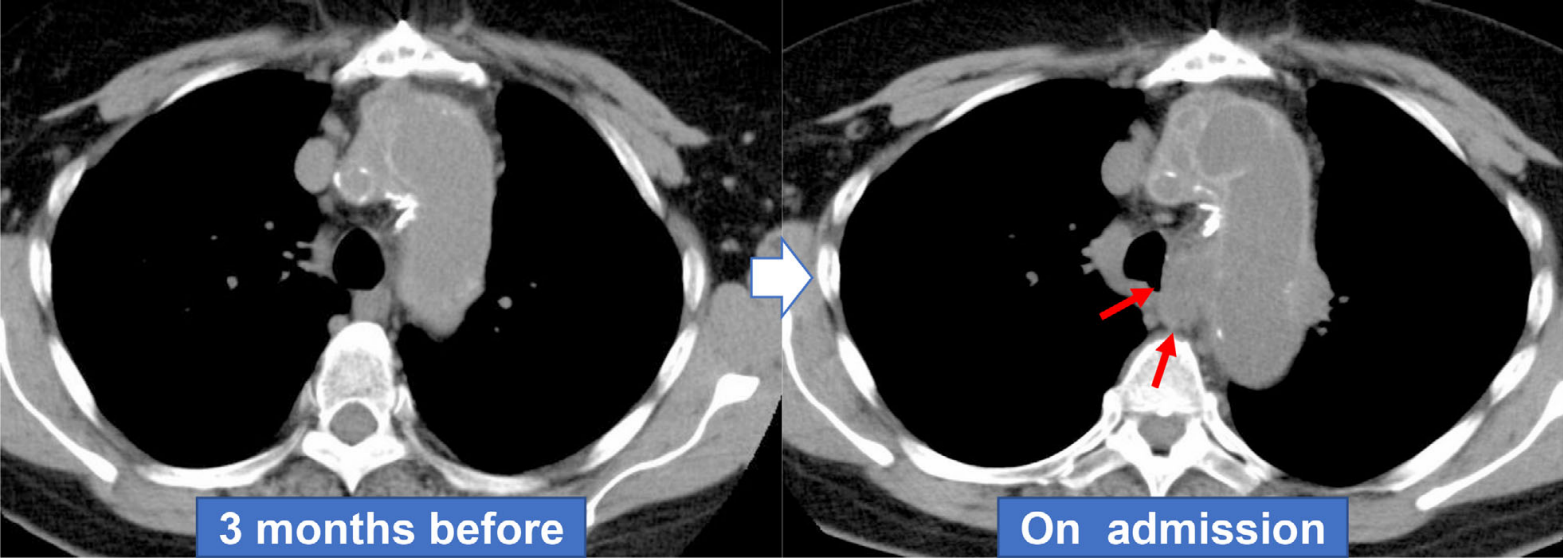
An abnormal mediastinal mass‐like lesion is observed in the para‐tracheal region on non‐contrast computed tomography (NCCT).
